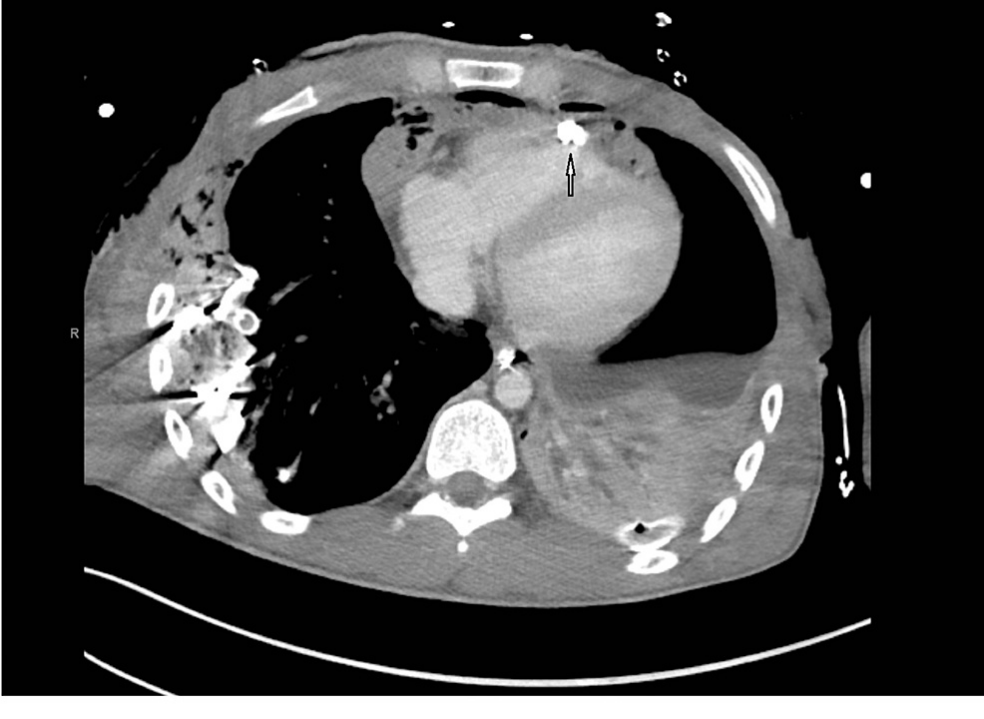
Computed Tomography (CT) Chest Obtained on Post-Operative Day 1 Figure 2 shows Computed tomography (CT) chest obtained on post-operative day 1, revealing missile (see arrow) lodged in the epicardial wall of the right ventricular outflow tract.
